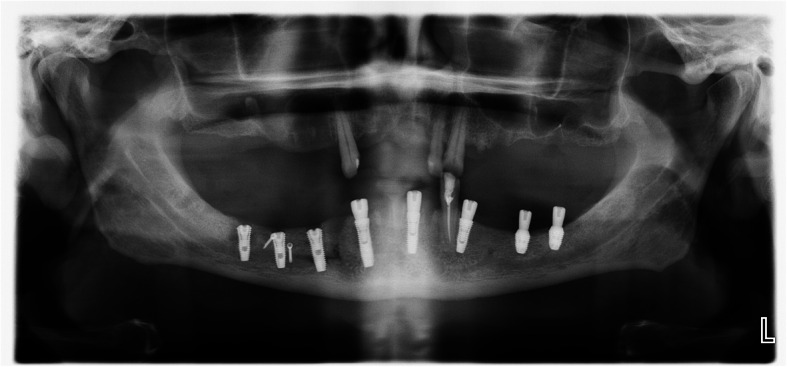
A panoramic radiography after IAN transposition and implant placement
